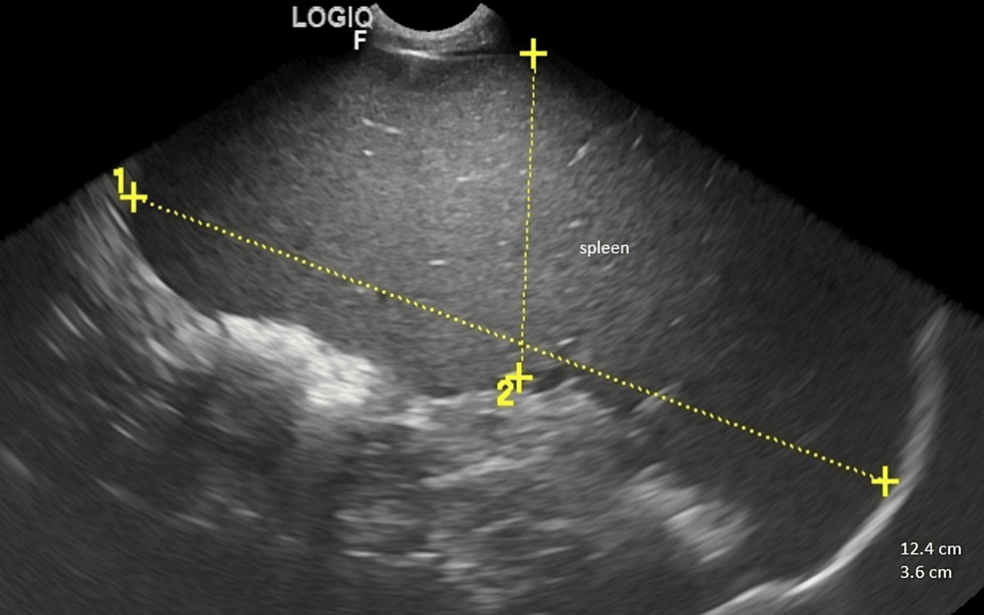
Ultrasonography revealed massive splenomegaly (12.4x3.6 cm, N<5x3 cm)
